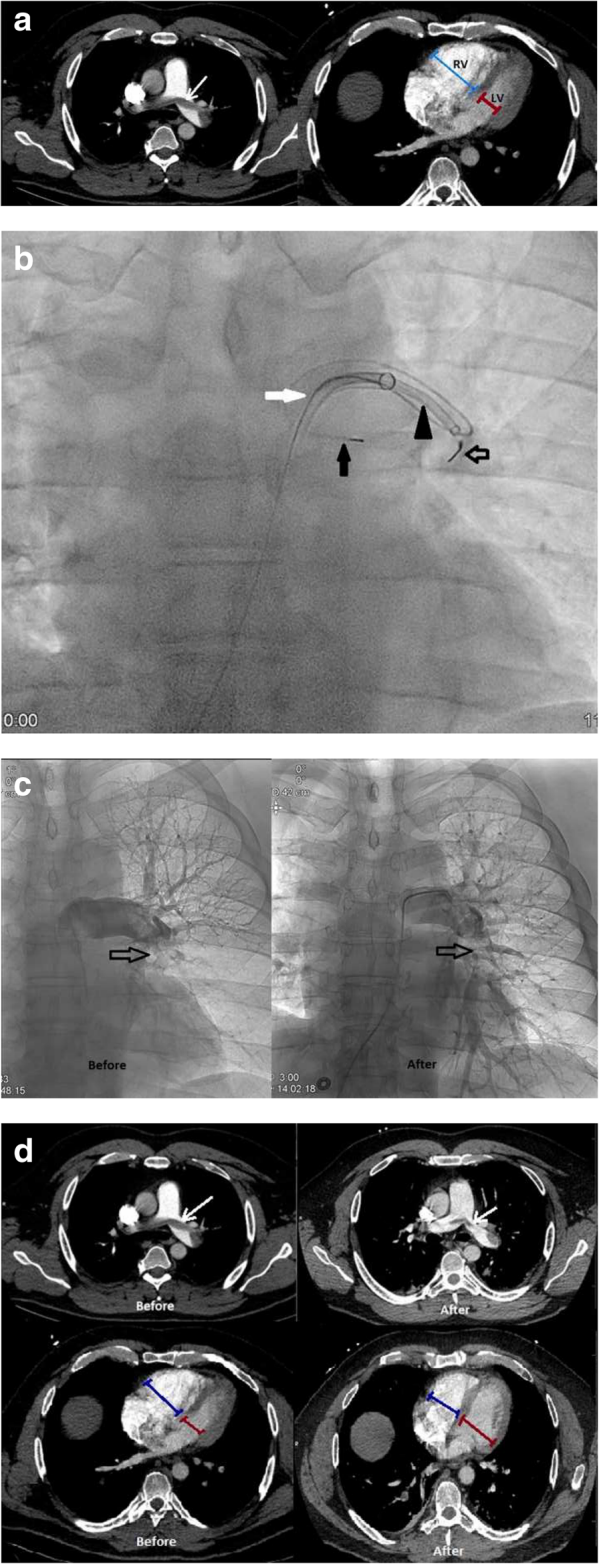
A 44-year-old male with a left lower extremity DVT after trauma presenting with massive PE, 8 days after initiation of anticoagulation for the DVT. Patient was hypotensive with a BP of 78/45 and troponin I elevation of 0.83 ng/mL. a CTA demonstrates a saddle pulmonary embolus (white arrow) and increased RV/LV ratio with blue calipers demonstrating the diameter of the RV and the red calipers demonstrating the diameter of the LV. b Fluoroscopic images of aspiration thrombectomy. A 90 cm 8Fr Sheath (white arrow) through which is passed an Indigo CAT-8 thrombectomy device (black arrowhead, Indigo, Penumbra, Alameda, CA, USA). Black closed arrow demonstrates a safety wire to stabilize access to the left pulmonary artery. Black open arrow demonstrates the Indigo separator to clear thrombus from the opening of the catheter. c Pulmonary angiogram demonstrates before and after catheter-directed therapy pictures. Arrow demonstrates improved perfusion to the lower lobe. d CTA demonstrates before and after catheter-directed therapy images with improvement in thrombus burden and before and after catheter-directed therapy images with improvement in RV/LV ratio
